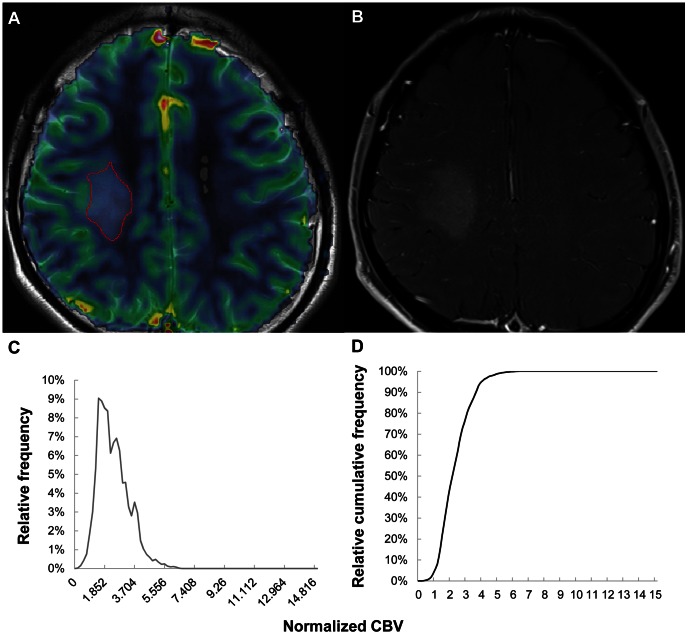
Images of a 35-year-old male with a grade III astrocytoma. (A) ROI-drawn rCBV map overlaid on a T2-weighted image, (B) contrast-enhanced T1-weighted image, (C) histogram of nCBV and (D) cumulative nCBV histogram. The 99^th^ percentile value of the cumulative nCBV histogram was 5.092. ROI = region of interest; rCBV = relative CBV; nCBV = normalized CBV.
